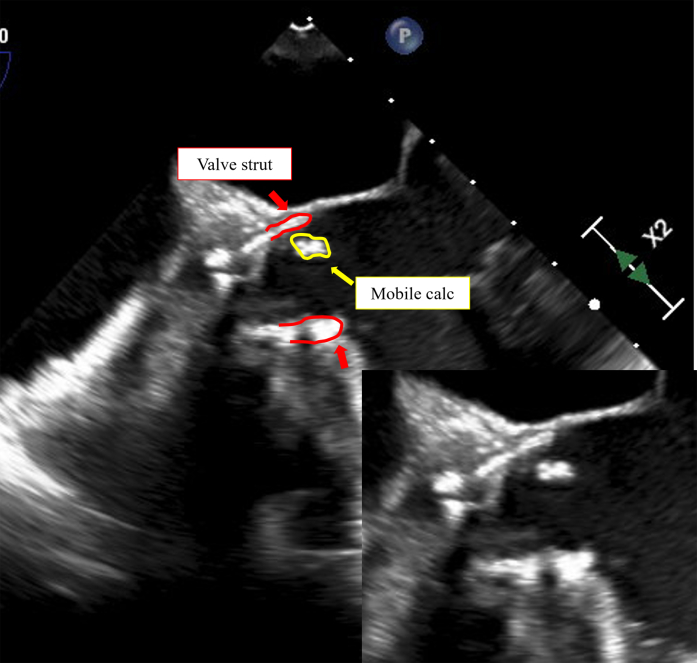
Transesophageal Echocardiogram During Percutaneous Coronary Intervention The area outlined in red indicates the valve strut, and the area outlined in yellow shows the mobile calcification located inside the strut. This is further demonstrated in Video 4.
